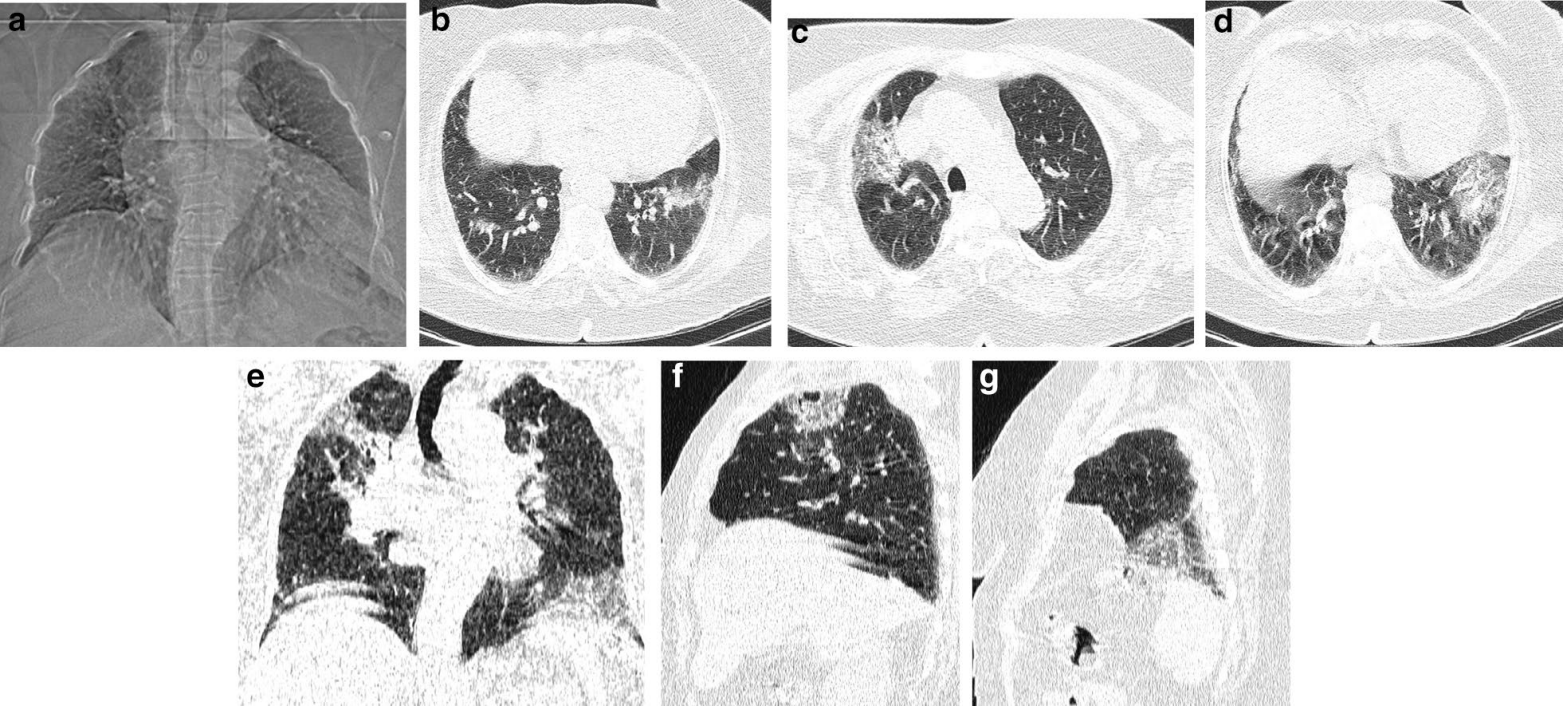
A 78-year-old, non-diabetic lady with heart failure, suggested by a large heart on topogram image (CT ratio > 60%) (A) and large intrapulmonary arteries (broncho-arterial ratio < 0.5) (B), showed mild lung involvement in her first-day in-hospital CT (C–G) with CTSS1 = 3/24, CTSS2 = 5/48, CTSS3 = 3/20, CTSS4 = 5/25, CTSS5 = 7/20, CTSS6 = 7/40, CTSS7 = 5/30. After 7 days in intensive care unit (ICU) (severe disease on triage), she got intubated and died after another day (critical disease on prognostication)
